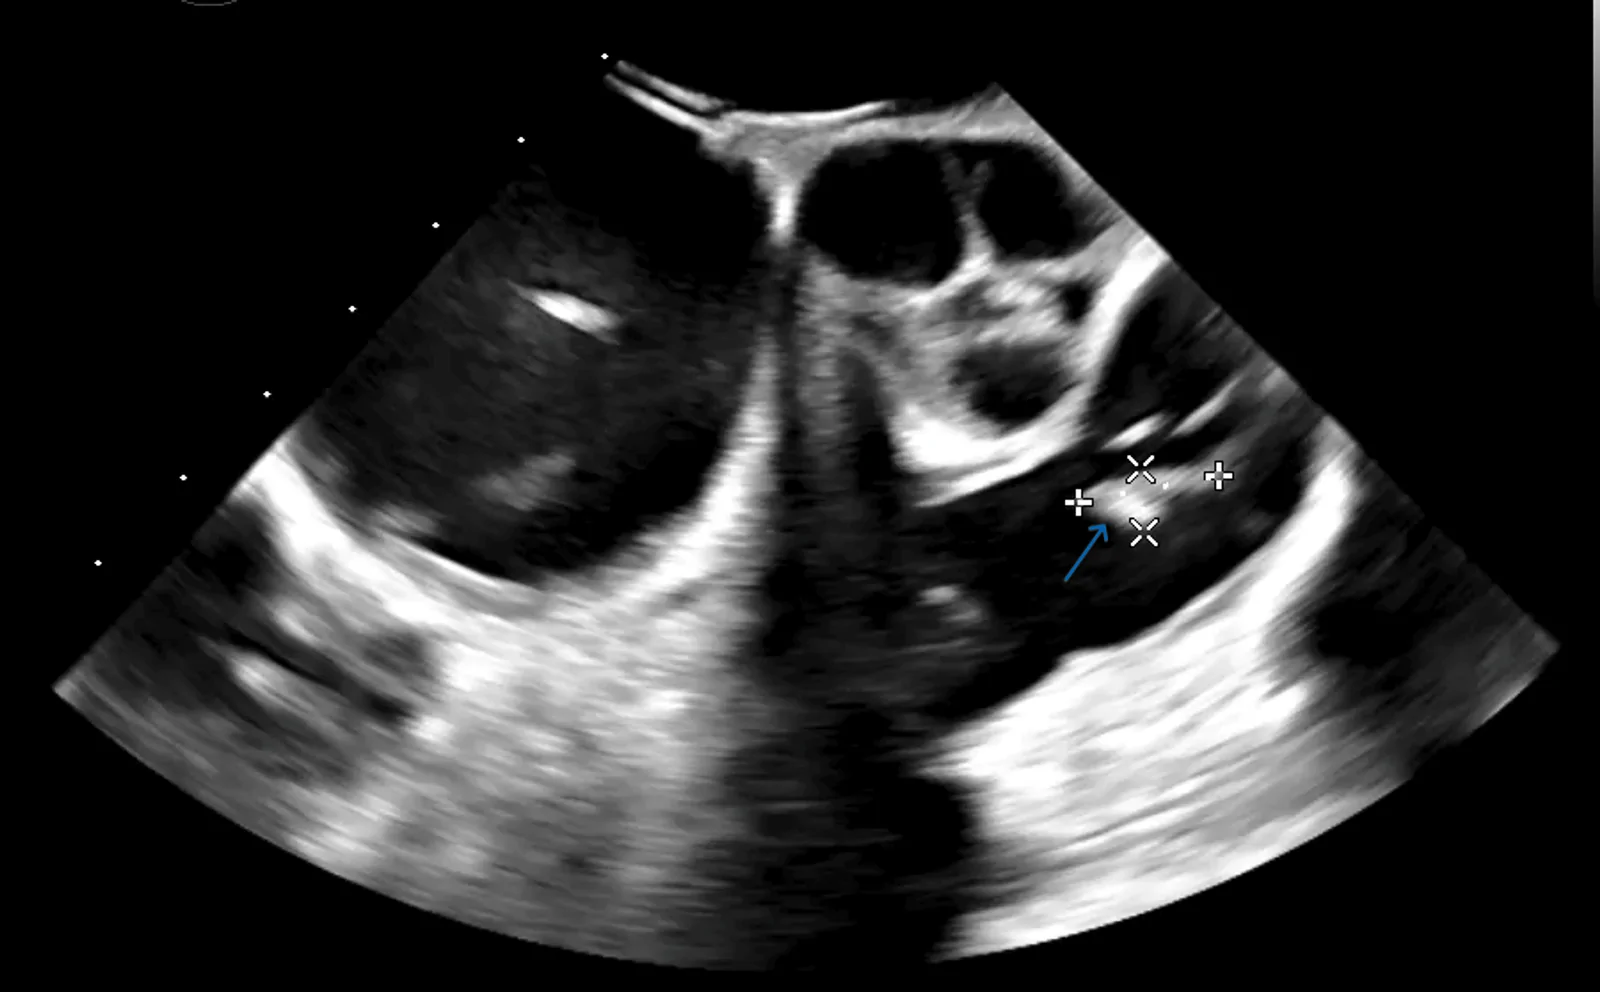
Transoesophageal echocardiogram (TOE) image demonstrating a mobile echogenic mass attached to the pulmonic valve (1.2 cm × 0.5 cm) (blue arrow) (mid-oesophageal right ventricular inflow-outflow view).
